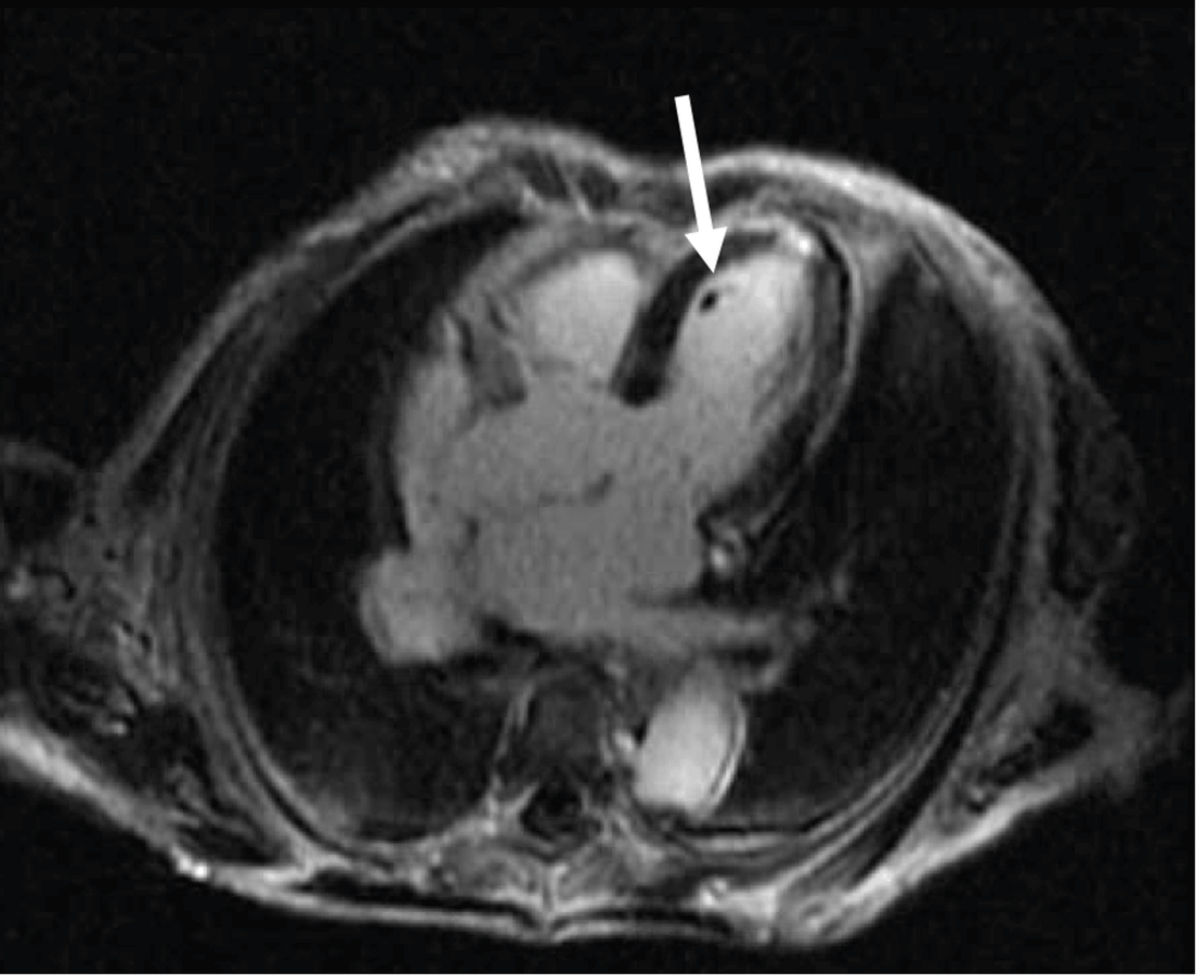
Cardiac magnetic resonance imaging in Case 3, revealing a discrete thrombus (white arrow) within the left ventricle.
